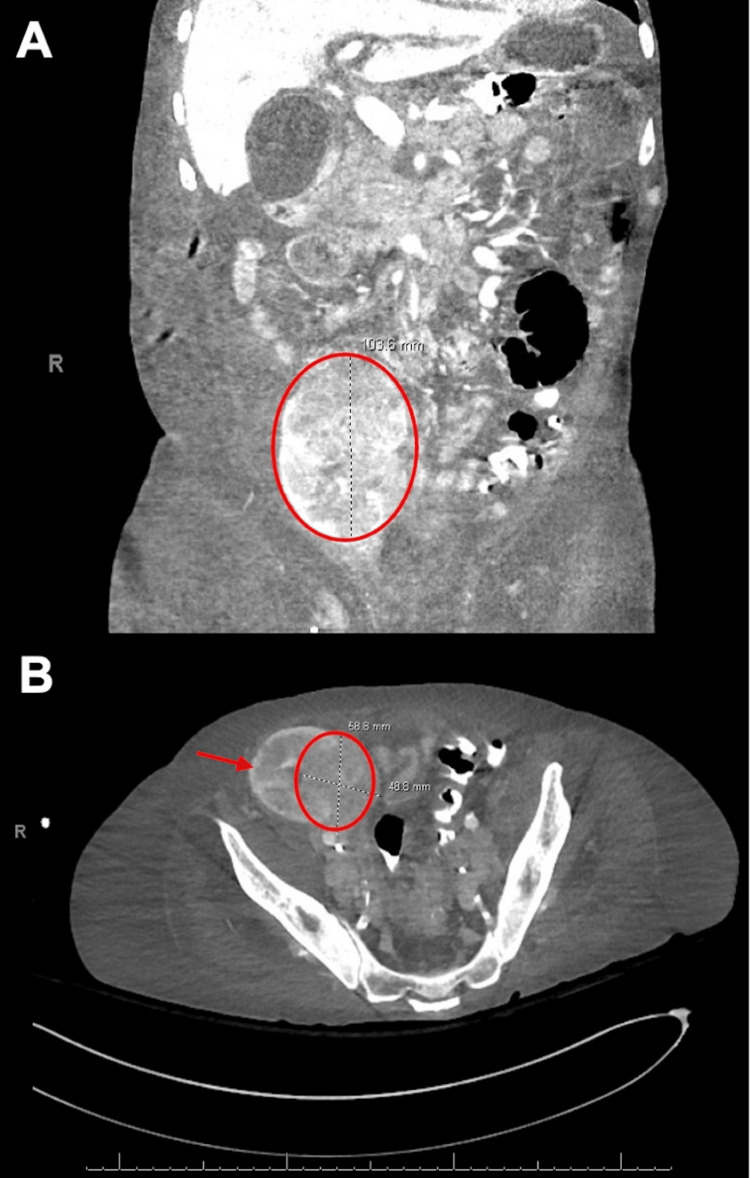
CT abdomen pelvis revealing neoplastic process
